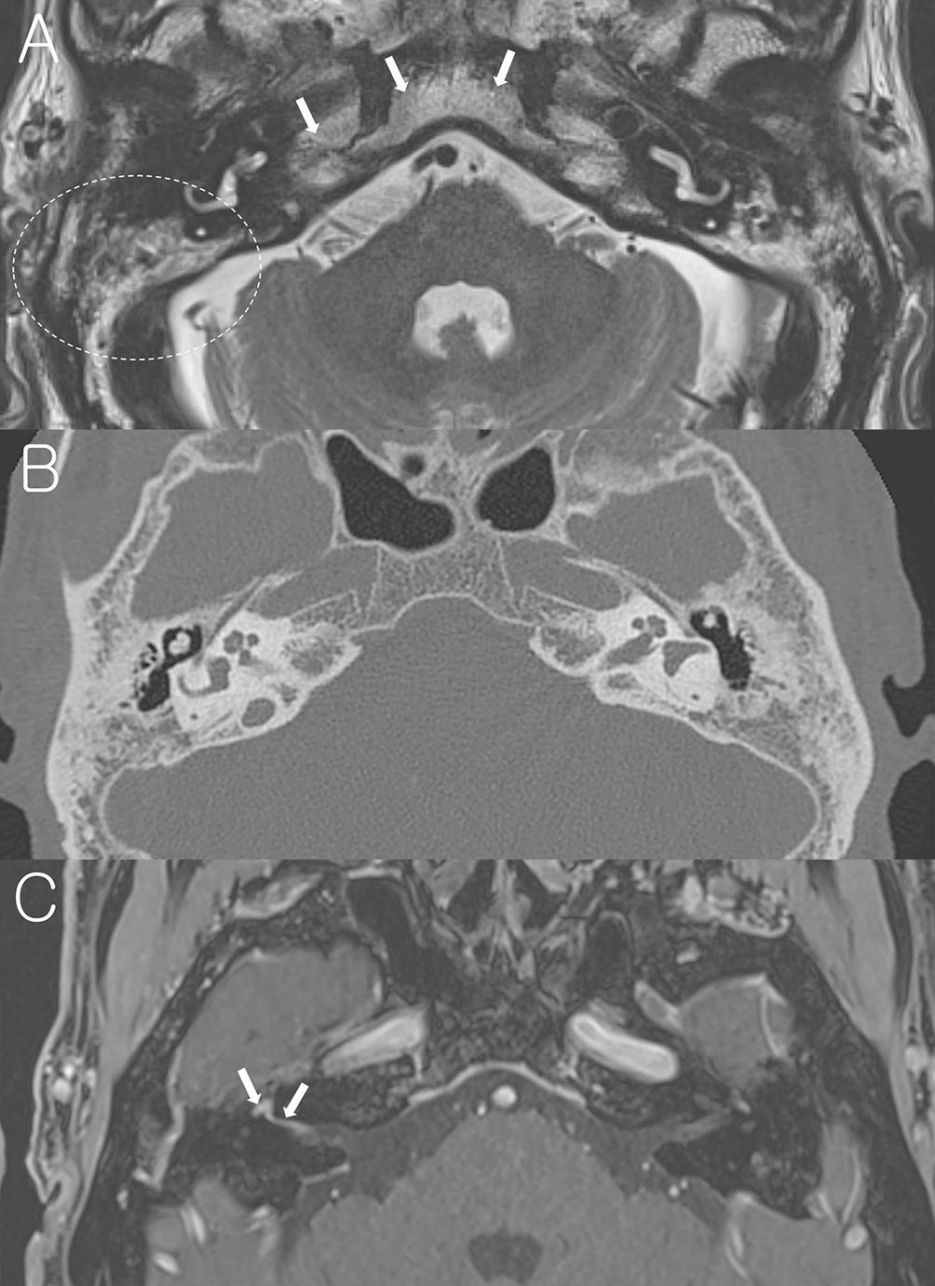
A representative case of Bell’s palsy with sclerotic mastoid. (A) Non-contrast axial T2-weighted image at the level of IAC shows heterogeneous high signal intensity in the mastoids (dotted circle) which have similar signal intensity from the adjacent clivus and petrous apex (arrows), suggesting bony sclerotic change. (B) Temporal bone CT confirms bony sclerotic change and decreased number of mastoid air cells. (C) Post-contrast axial T1-weighted image at the Bill’s bar shows enhancement of the distal canalicular, labyrinthine, genu and proximal tympanic segment of the right facial nerve (arrows).
